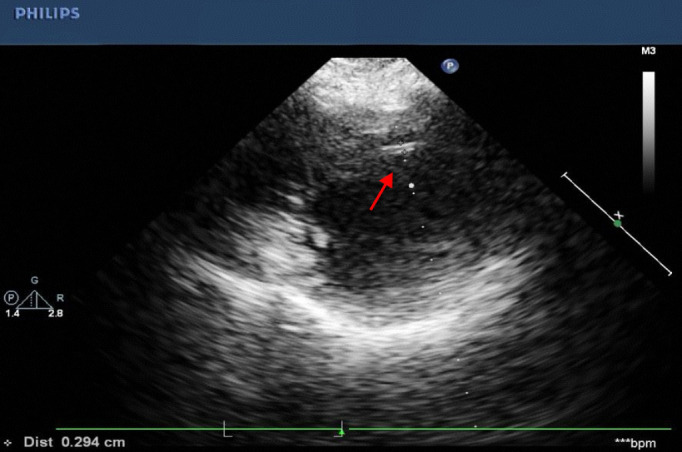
Follow-up echocardiogram at 2 months. The study shows reduction in the thickness of the septal endocardium to 2.94 mm (arrow), indicating response to treatment.
